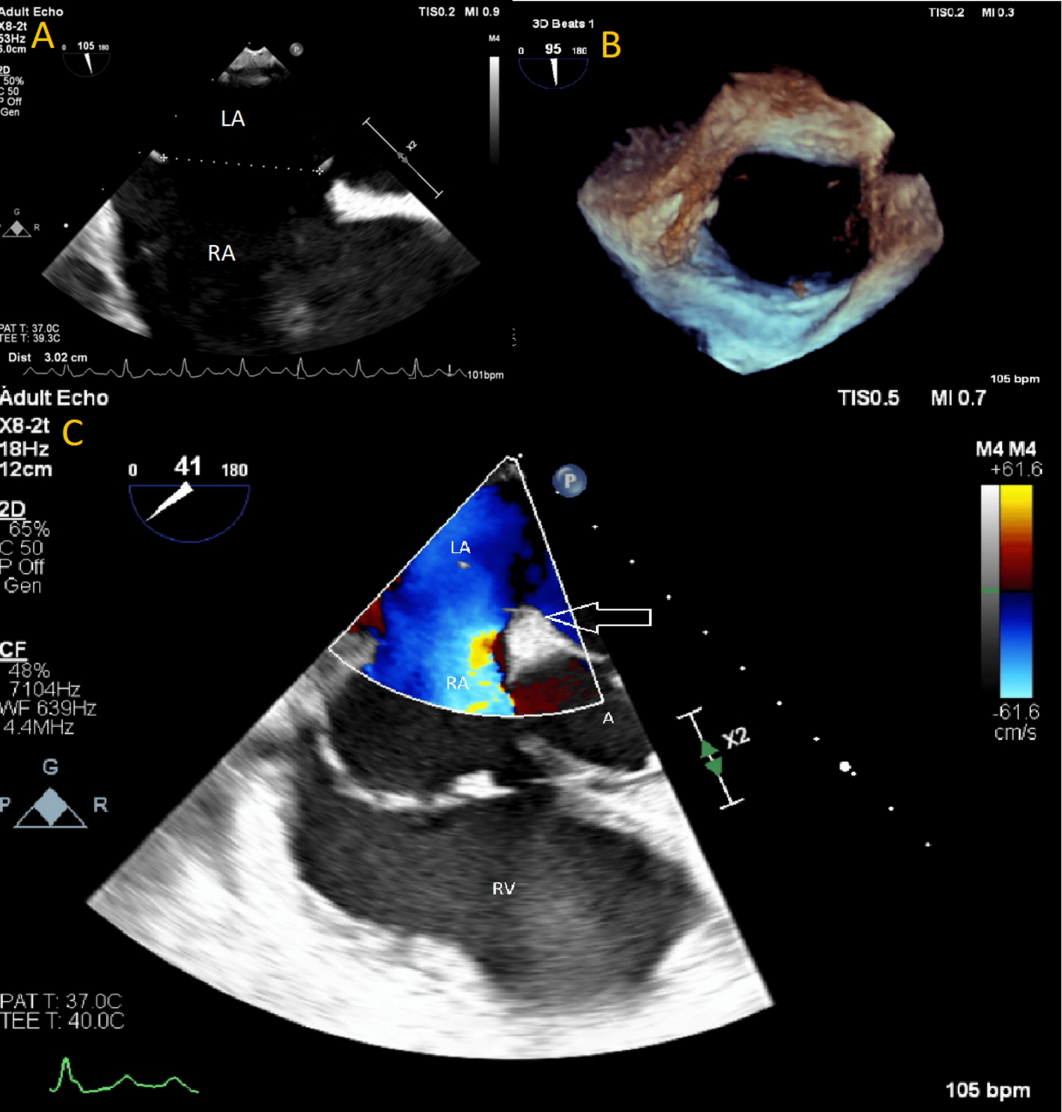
TEE images. A) ASD measuring 3 cm; B) 3D reconstruction of the ASD; C) TEE with color Doppler demonstrating left to right shunt LA: left atrium; RA: right atrium; RV: right ventricle; A: aorta; ASD: atrial septal defects; TEE: transesophageal echocardiogram
